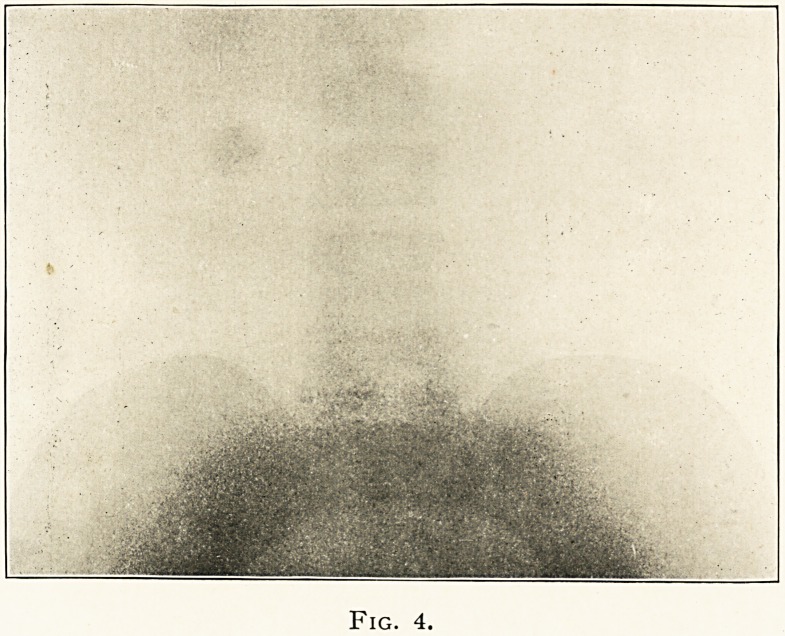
# The Use of X-Rays in the Diagnosis of Renal Calculi
